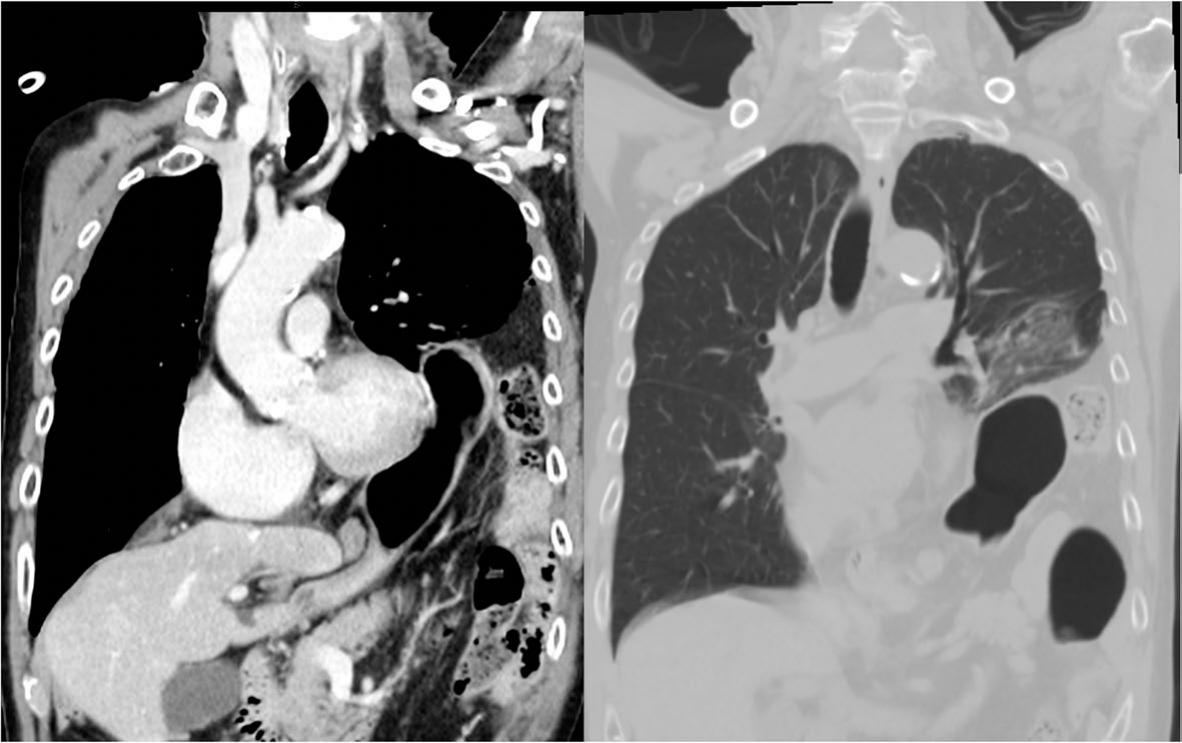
CT images at consultation. A herniation of the stomach, the small intestine, and the colon through into the left thoracic cavity and compressed lung was observed
